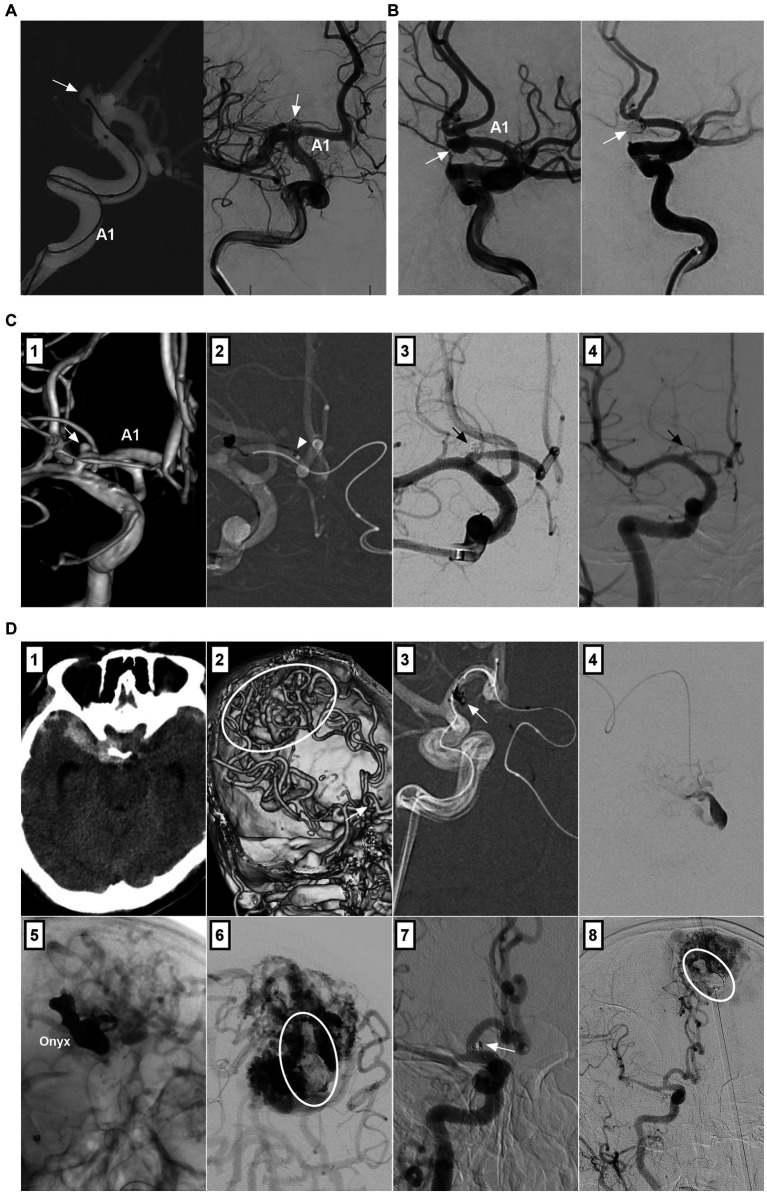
Treatment of A1 aneurysms by traditional coiling. (A) Left panel: Roadmap image showing the straight microcatheter inserted into the A1 aneurysm (arrow); Right panel: DSA showing the A1 aneurysm (arrow) after coiling. (B) Left panel: DSA showing an aneurysm (arrow) at A1 termination; Right panel: DSA showing the aneurysm (arrow) after coiling. (C) Panel 1: 3D-DSA showing an A1 aneurysm (arrow); Panel 2: Roadmap image showing the A1 aneurysm coiled via contralateral approach; the arrowhead indicates the distal marker of the stent; Panel 3: Postoperative immediate DSA showing the A1 aneurysm (arrow) after coiling; Panel 4: Six-month follow-up DSA showing that the A1 aneurysm (arrow) had no recurrence. (D) Panel 1: CT showing subarachnoid hemorrhage; Panel 2: CTA showing an aneurysm (arrow) at A1 segment and an AVM (circle); Panel 3: Roadmap image showing the A1 aneurysm (arrow) coiled via contralateral approach; Panel 4: Selective microcatheter angiography showing the aneurysmal structure in the AVM; Panels 5 and 6: DSA showing Onyx casting (circle) in aneurysmal structure; Panel 7: Six-month follow-up DSA showing that the A1 aneurysm (arrow) had no recurrence; Panel 8: DSA showing no recurrence of the aneurysmal structure in the AVM (circle). 3D, three-dimensional; A1, first segment; ACA, anterior cerebral artery; AVM, arteriovenous malformation; CT, computed tomography; CTA, computed tomography angiography; DSA, digital subtracted angiography.
